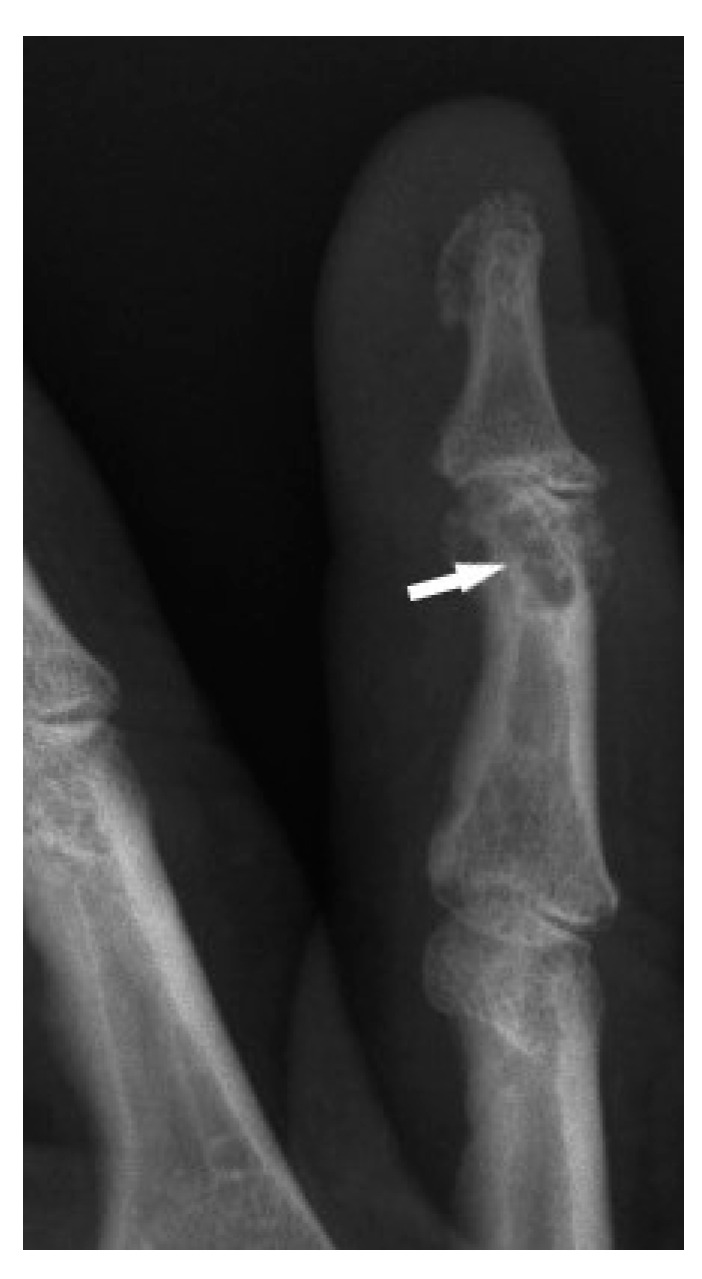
X-ray of the finger. Sarcoidosis. Cystic lesions in the middle phalanx head (white arrow).
